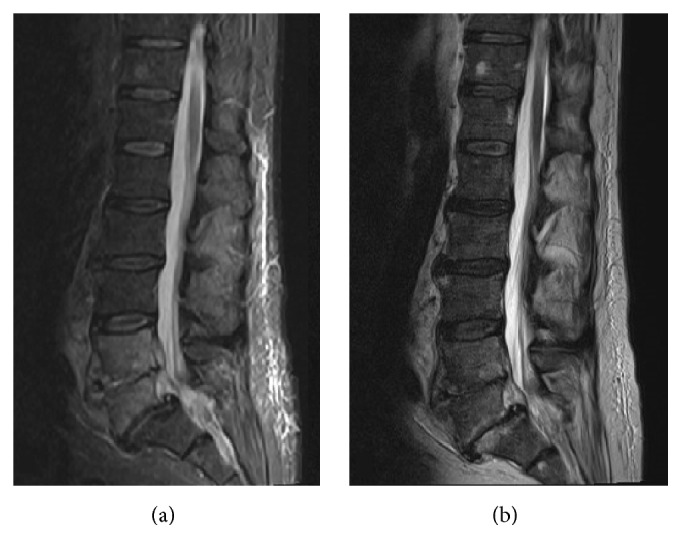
Lumbar spine MRI scan. (a) STIR sequence; (b) T2 sequence.
